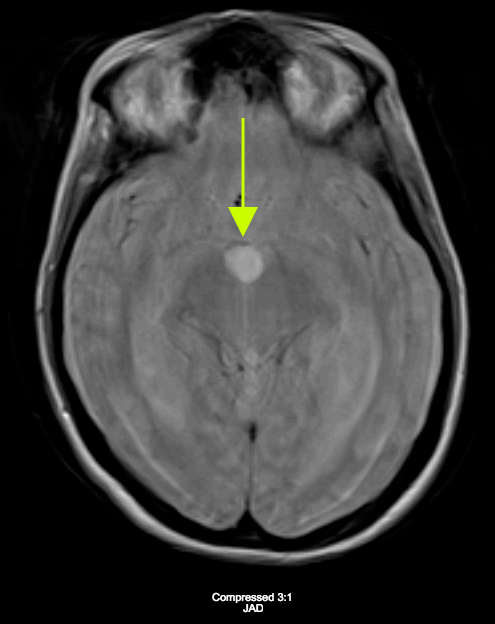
MRI of the Brain without Contrast B: FLAIR image displaying hyperintense signal at the Foramen of Monro consistent with colloid cyst.
